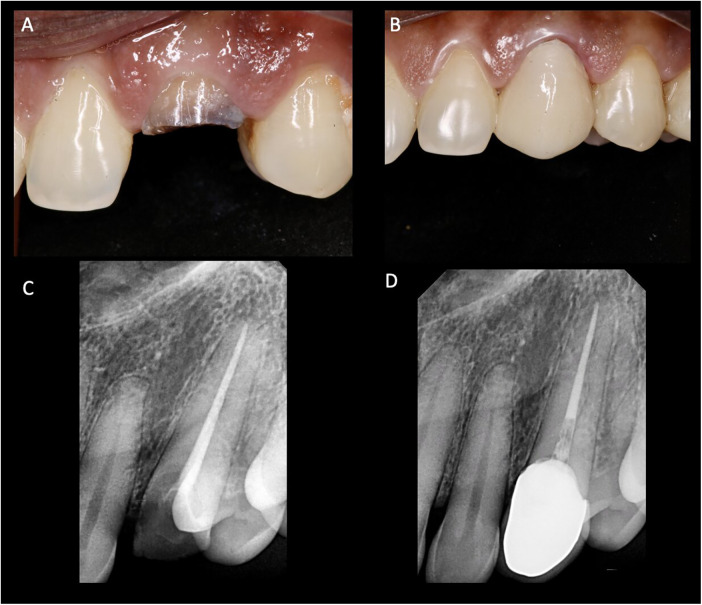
Comparative preoperative and postoperative clinical and radiographic outcomes. (A) Preoperative clinical view. (B) Postoperative clinical view after definitive rehabilitation. (C) Preoperative intraoral periapical radiograph. (D) Postoperative intraoral periapical radiograph showing satisfactory post placement and definitive restoration.
